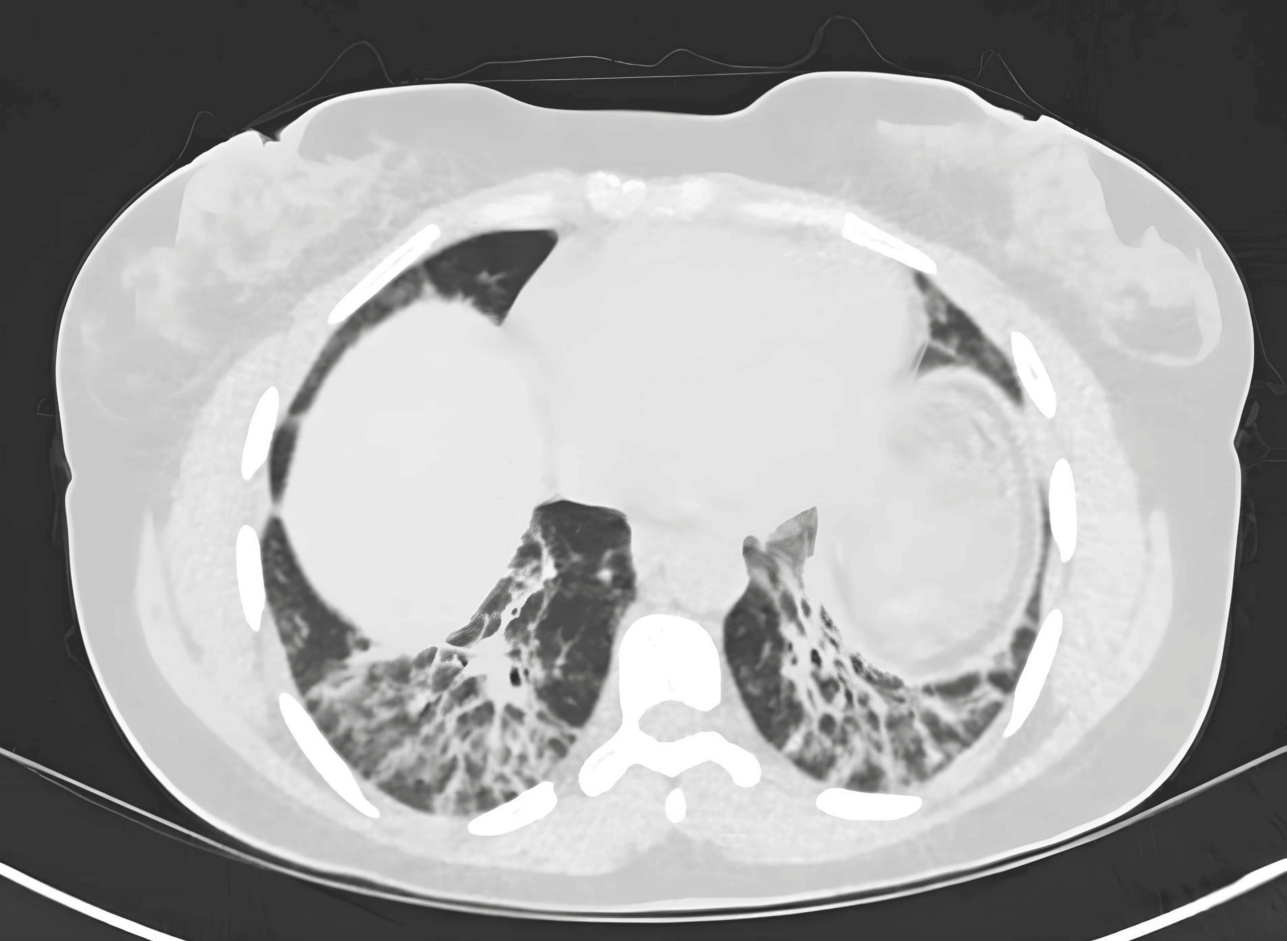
CT Thorax is showing bilateral patchy consolidation with ground-glass opacities, suggesting differential diagnoses of organizing pneumonia, chronic eosinophilic pneumonia, COVID-19, or atypical pneumonia. CT = Computed Tomography
